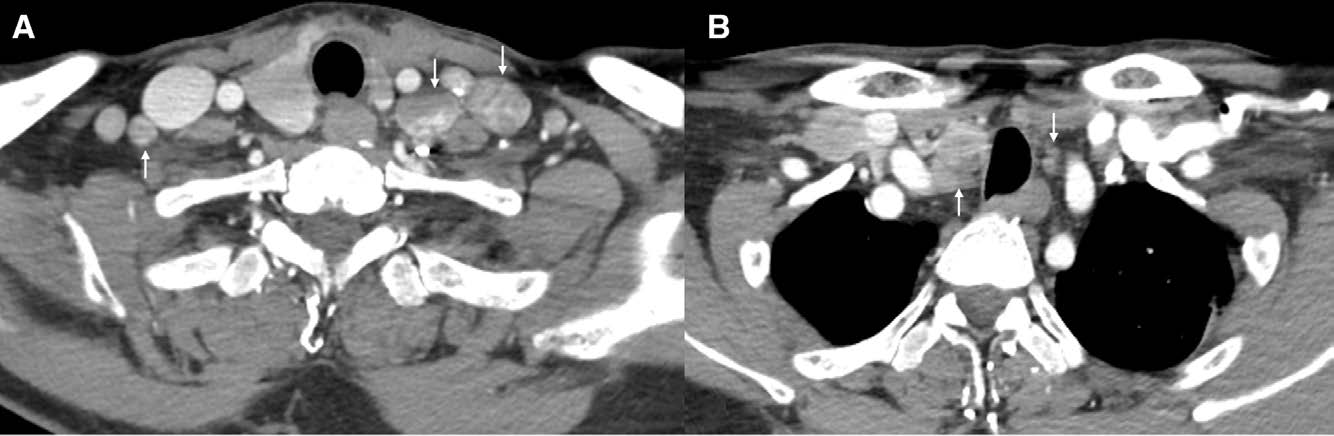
Computed tomography revealed heterogeneously enhanced lymph node enlargements (arrows) in the bilateral level IV (A) and VI (B) regions.
